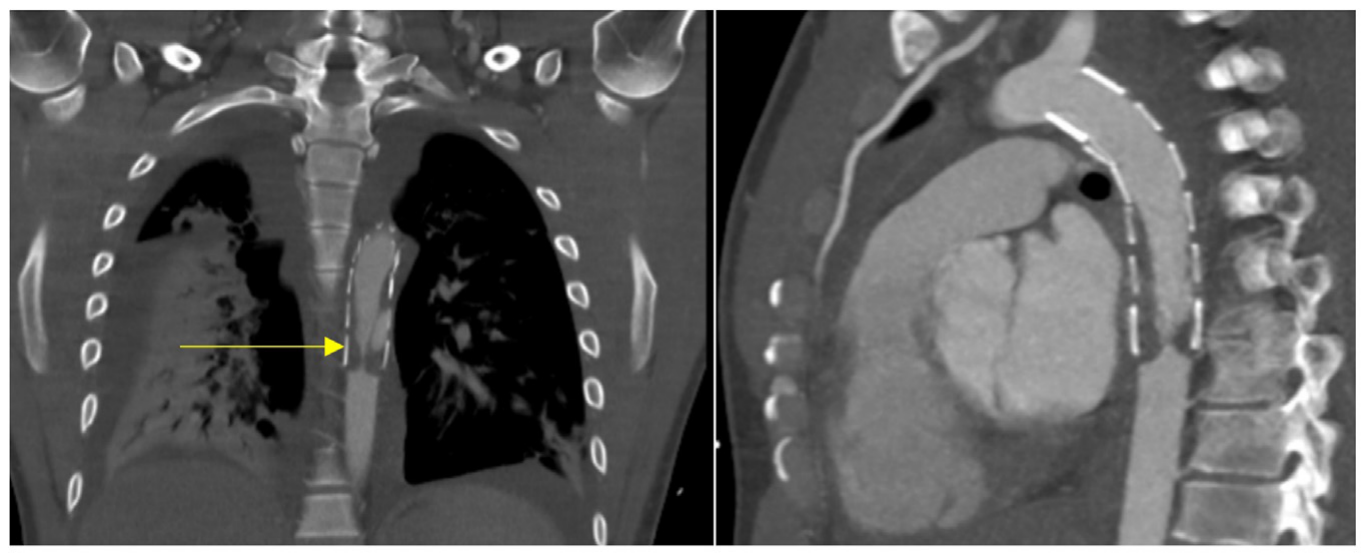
Computed tomography angiography (CTA) revealing circular wall thrombus of distal part of thoracic aortic endograft (*yellow arrow*) resulting in subtotal occlusion. Bilateral lung consolidation consistent with coronavirus disease 2019 (COVID-19) infection also visible.
